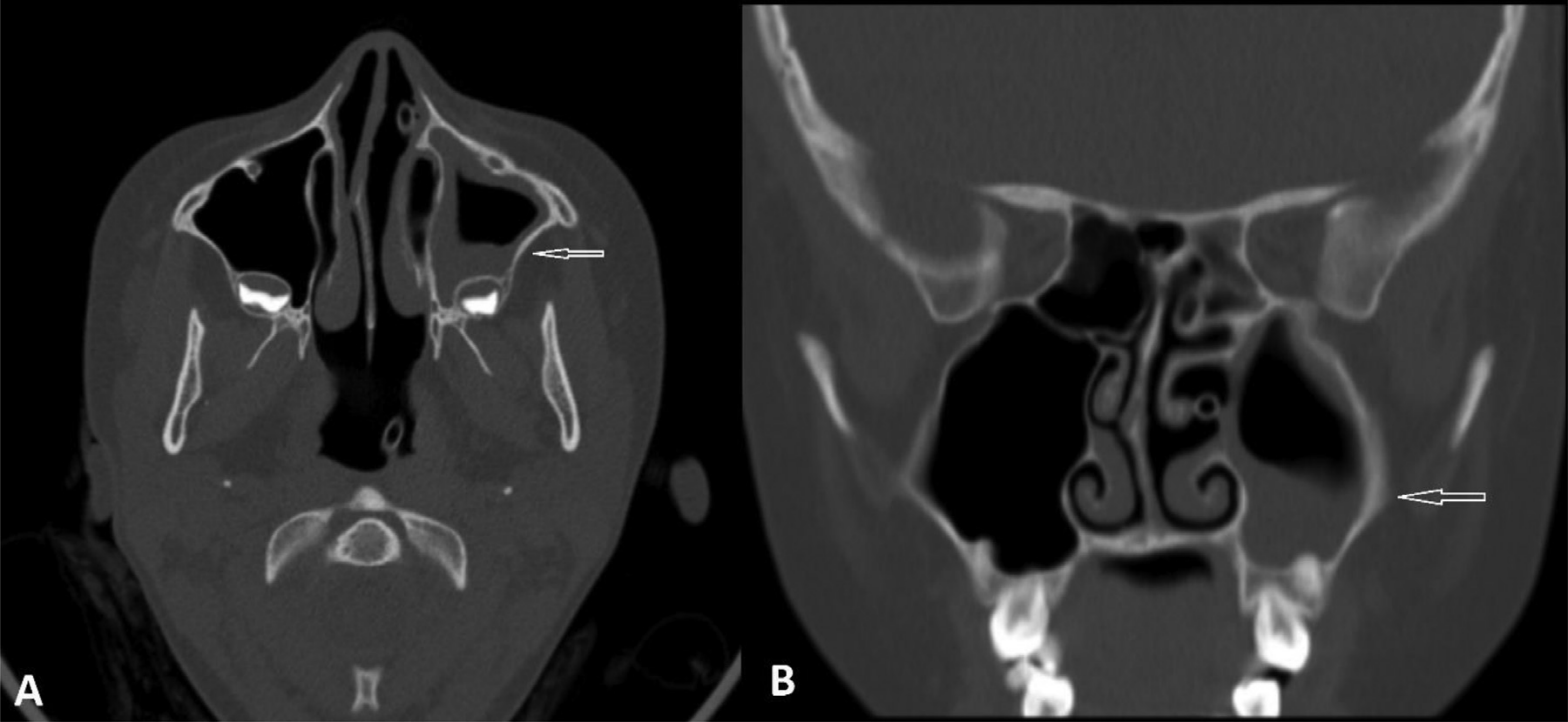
Axial (A) and coronal (B) paranasal sinuses CT scan without contrast: evidence of left maxillary sinus mucosal thickening is noted (arrows).
